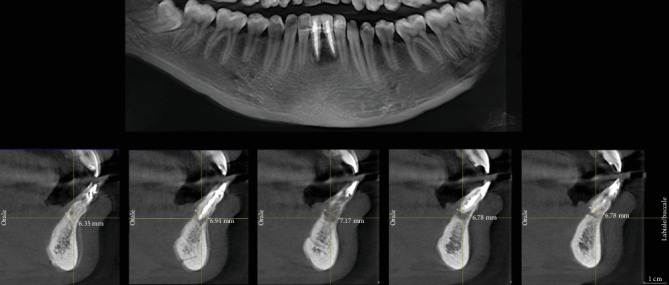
Radiographs of preoperative situation.
